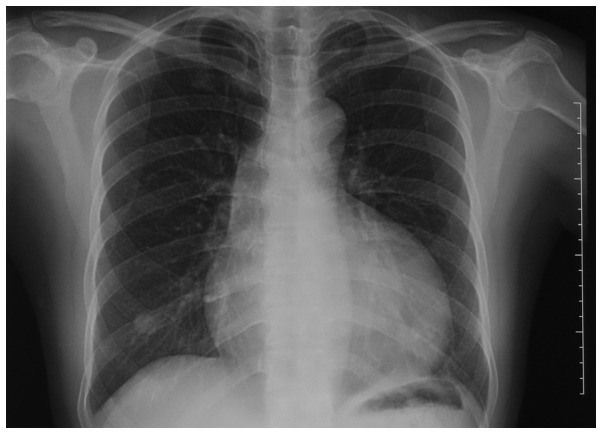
Chest X-ray reveals an enlargement of the cardiac silhouette. The cardiothoracic ratio is 64%.
